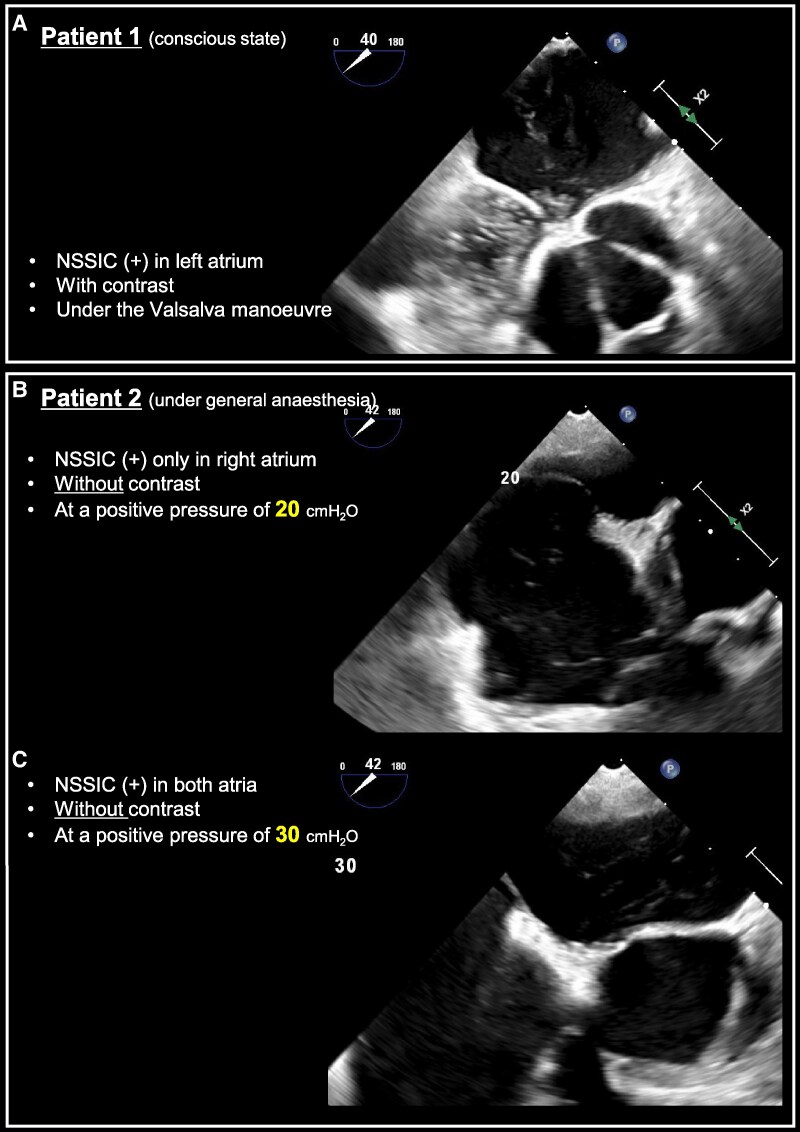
A NSSIC-positive case during microbubble testing in TOE without PFO (*A*). (*B* and *C*) TOE performed under general anaesthesia in a patient undergoing percutaneous PFO closure, using 10 s of positive pressure ventilation without contrast bubbles. In *B*, positive pressure ventilation at 20 cmH₂O induced NSSIC in the right atrium only, whereas in *C*, ventilation at 30 cmH₂O resulted in NSSIC being observed in both the right and left atria. NSSIC, non-smoke spontaneous individual contrast; TOE, transoesophageal echocardiography; PFO, patent foramen ovale.
